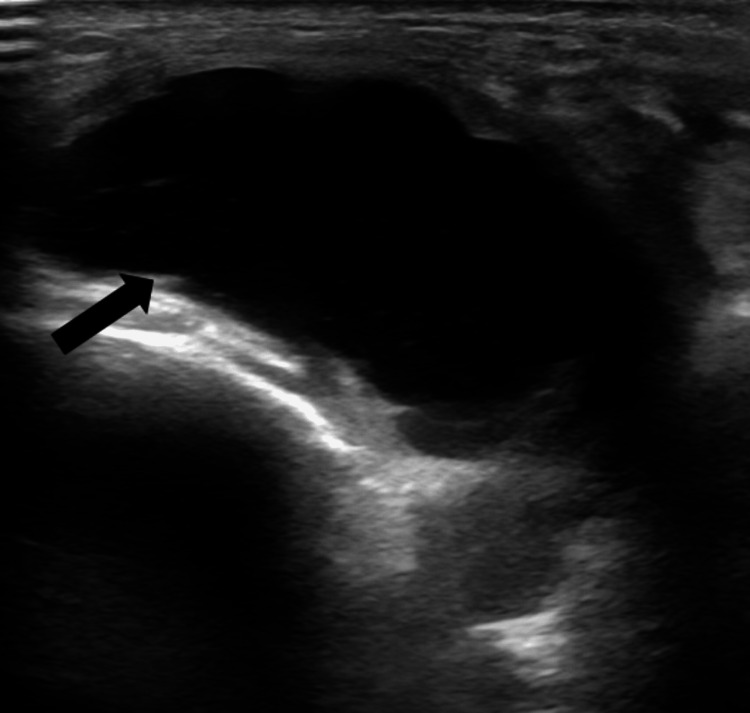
Repeat postoperative ultrasound of the right lower extremity demonstrated the recurrence of the intraneural cyst (black arrow) of the common peroneal nerve at the level of the fibular neck; additionally, the intraneural cyst can be seen in continuity with the deep peroneal nerve
